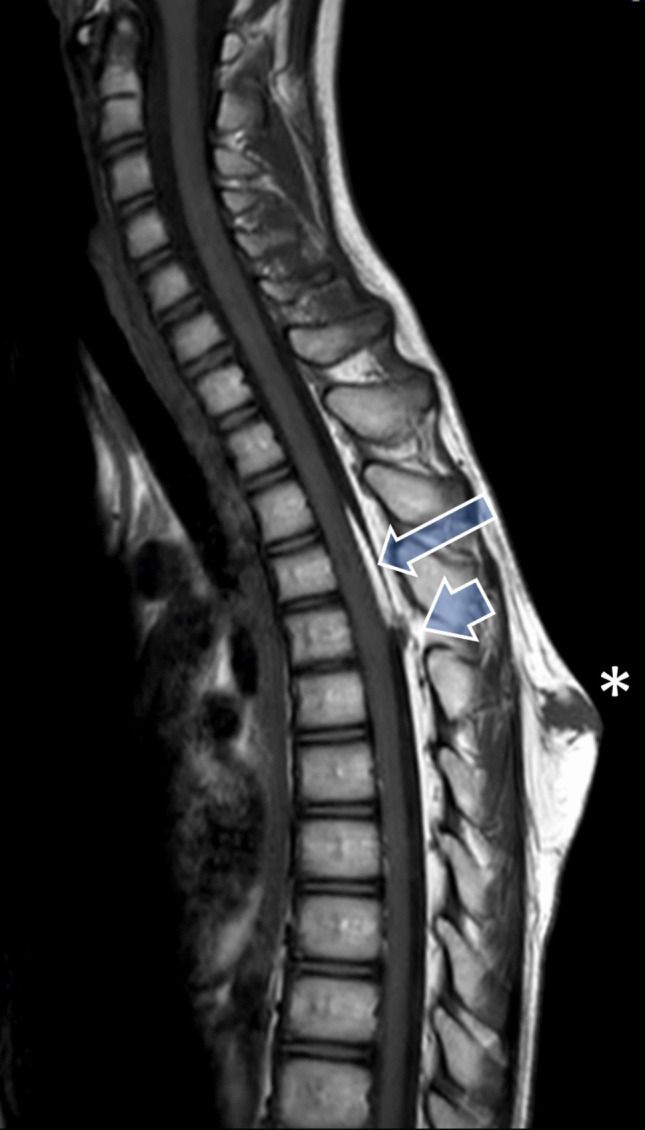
Spinal MRI findings: Sagittal T1-weighted MRI showing an intradural lipoma (*long arrow*) at the level T4 associated with a fibrous tract (*short arrow*) extending from the spinal canal toward the dorsal cutaneous lesion (*asterisk*), producing a tethered cord configuration
